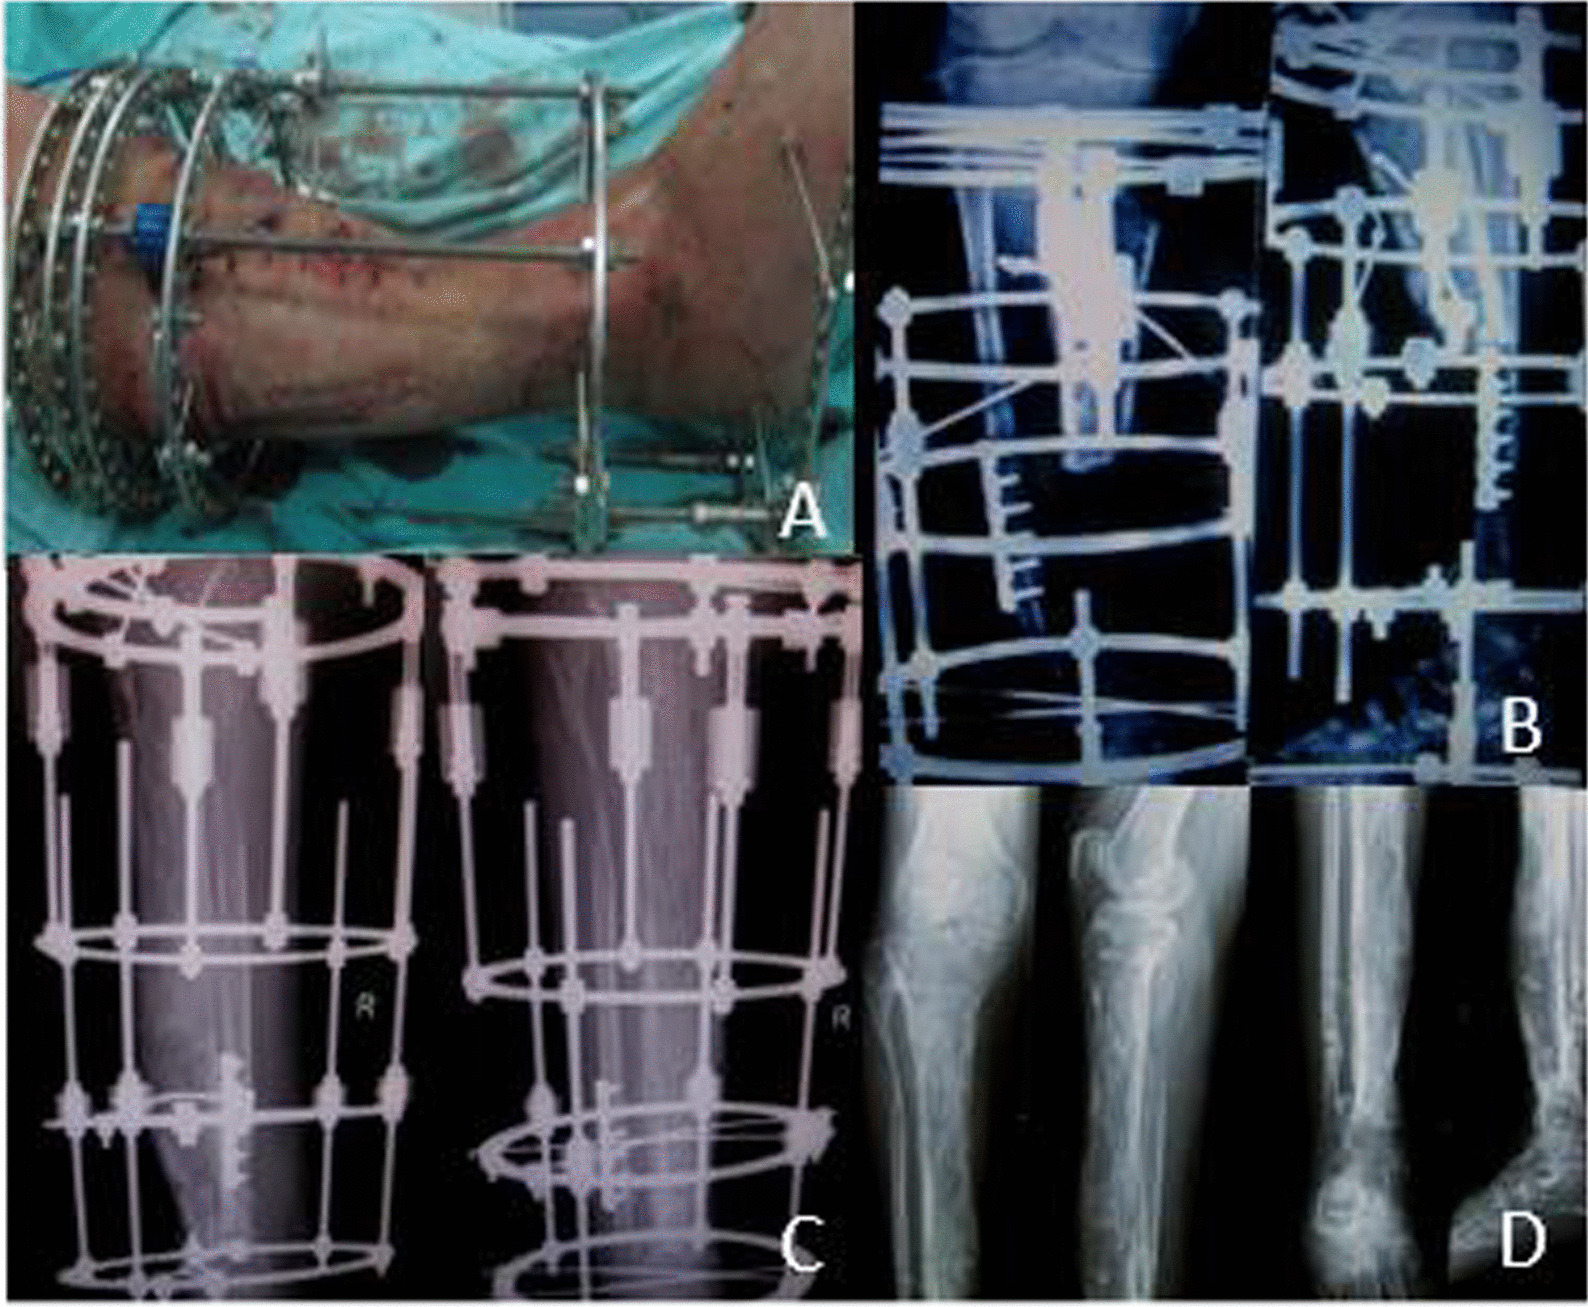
Imaging method was used to evaluate the prognosis of the patients. A Most of the wound was closed during shortening-lengthening operation. B Postoperative radiograph showed a good external fixation. C, D Anteroposterior and lateral X-ray film at 12 months after operation, and after the removal of fixators
